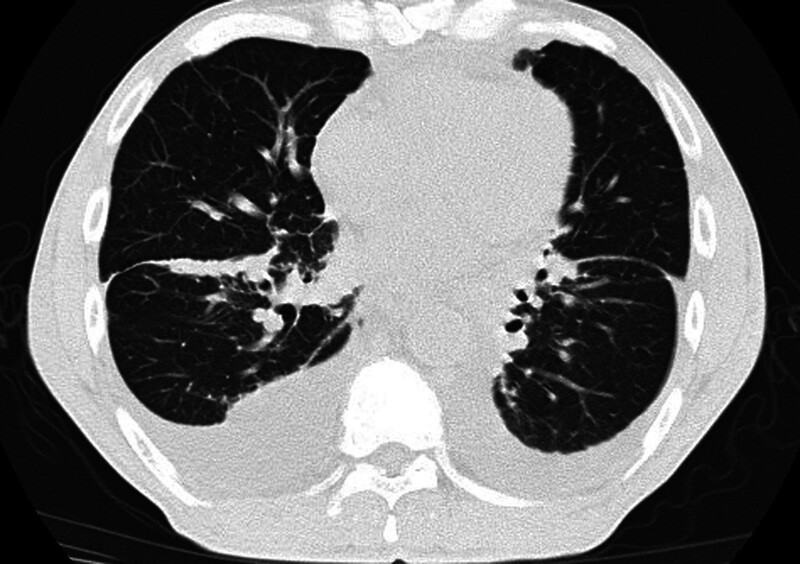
Chest CT scan-axial view.
